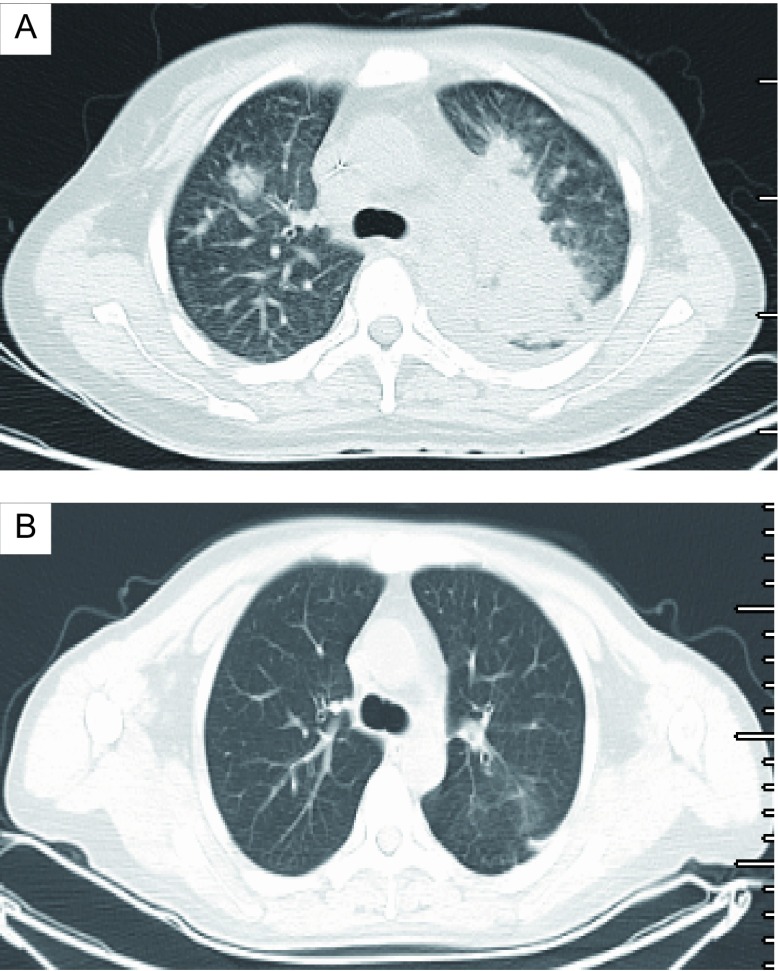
患者临床影像学特征。A：患者接受crizotinib治疗前，胸部CT显示左肺巨大占位，少量胸腔积液，右肺上叶可见团片状阴影，纵隔内可见肿大淋巴结；B：患者接受crizotinib治疗6周后，复查胸部CT显示左肺巨大占位明显缩小，胸腔积液消失，右肺上叶片状影消失，纵隔内淋巴结明显缩小。 Clinical radiologic features of the patient. A: Before crizotinib treatment, chest CT showing huge mass lesion in left lung and minimal pleural ef fusion, patchy shadows was seen in upper lobe of right lung and lymphonodus in mediastinum; B: After 6 wk of crizotinib, CT showing obvious shrink of left lung mass and lymphonomus in mediastinum, disappeared of pleural effusion and shadow in right lung. CT: computed tomography.
